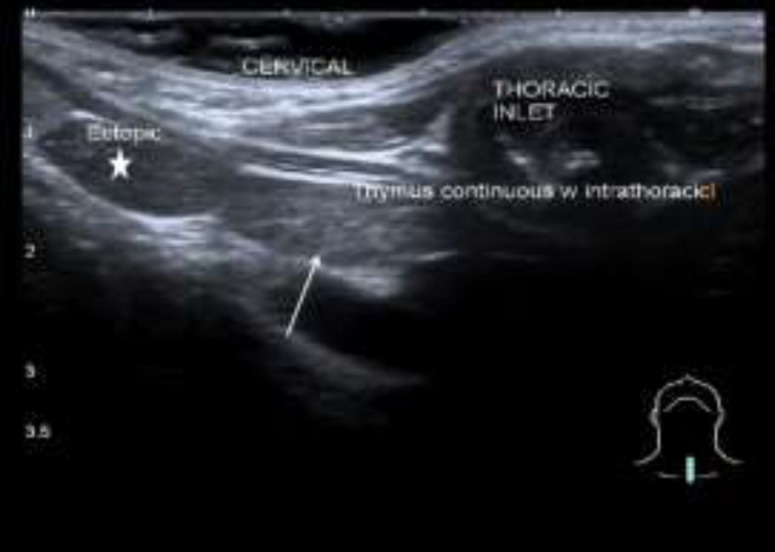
Ultrasound neck in sagittal plane at the level of suprasternal region shows the intrathoracic thymus (white arrows) is continuous with a portion of thymus that dynamically seen slides into suprasternal region upon Valsalva maneuver. A separated ectopic thymus (white star) noted more cranially in the cervical region
